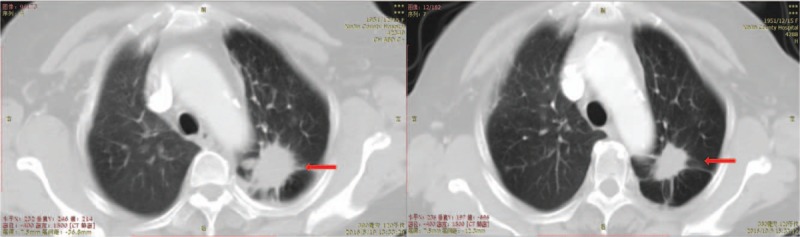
Computed tomography shows the mass in the left lung on lung window before and after treatment.
